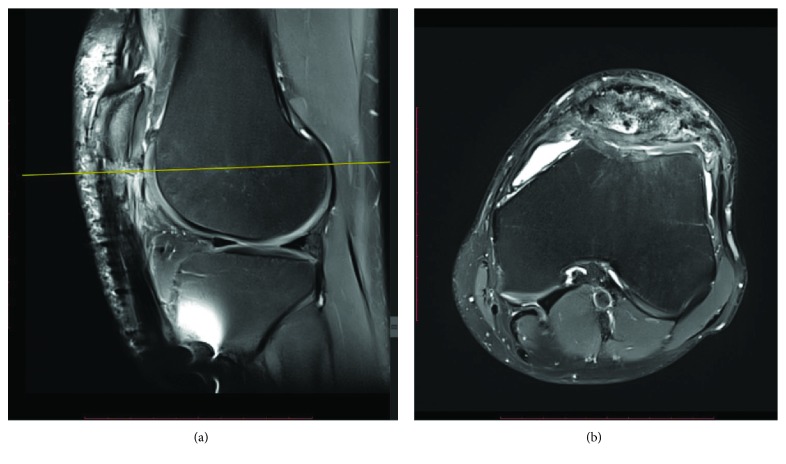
(a) T2 sagittal slice and (b) T2 axial slices of the control MRI at 1-year follow-up. The axial slice corresponds to the level of the fracture of the transverse patellar tunnel. Fracture is healed and continuity of the patellar tendon is preserved.
